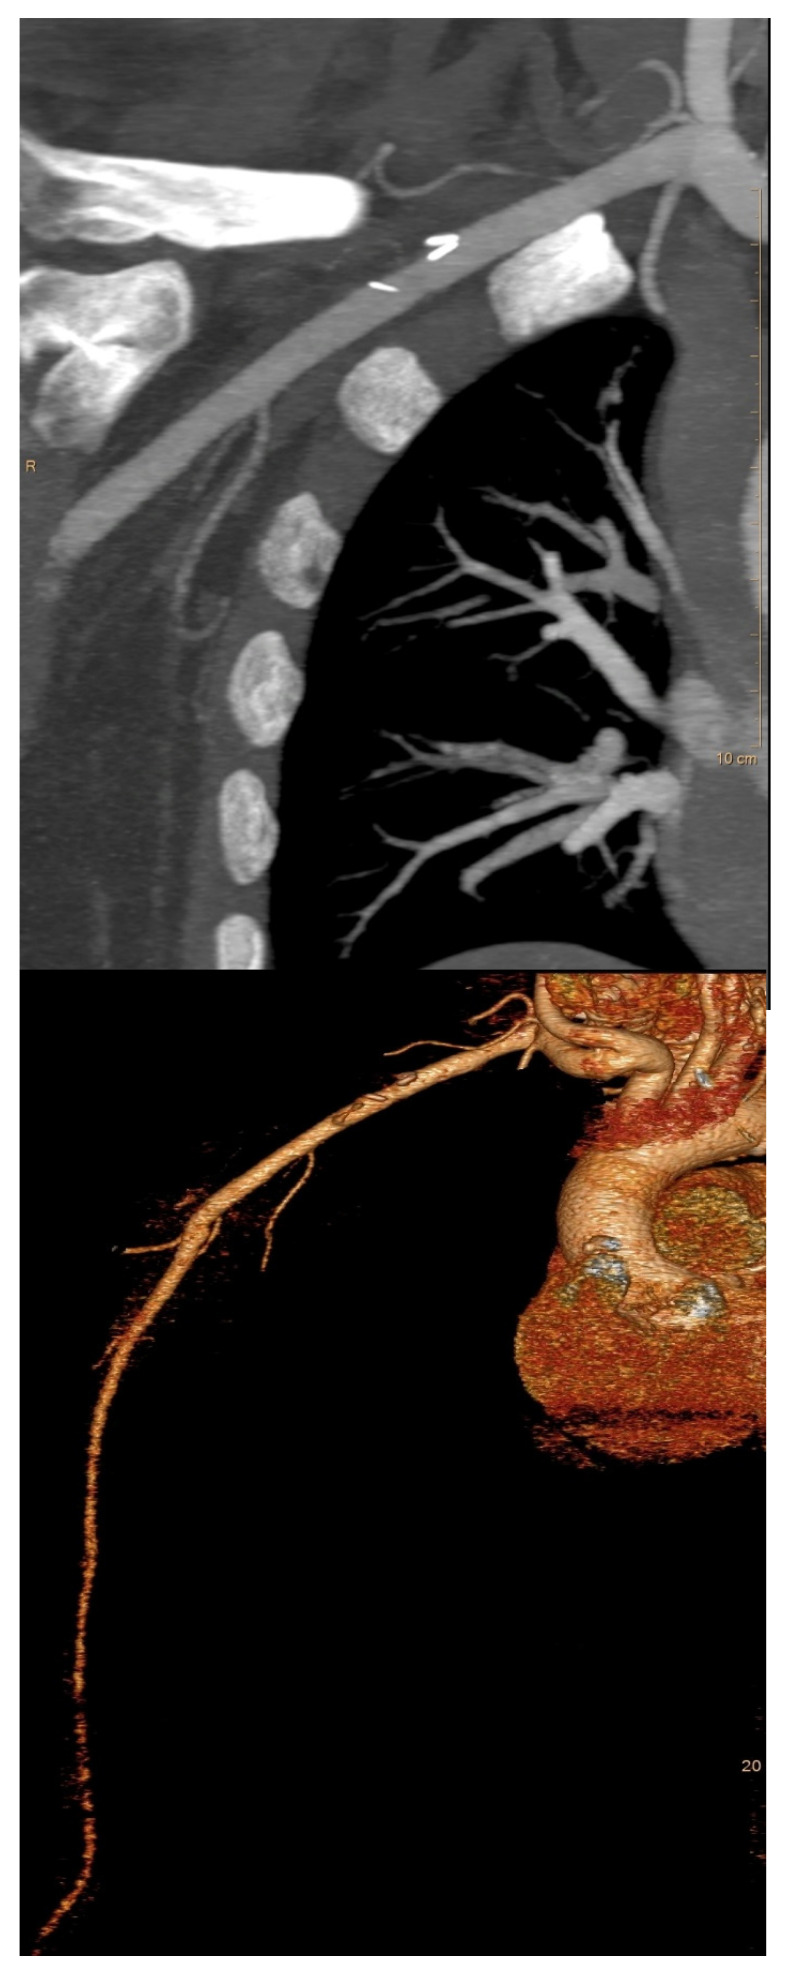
Postoperative CT scan after axillary cannulation and venous patch repair shows no stenosis of the vessel lumen after axillary cannulation.
